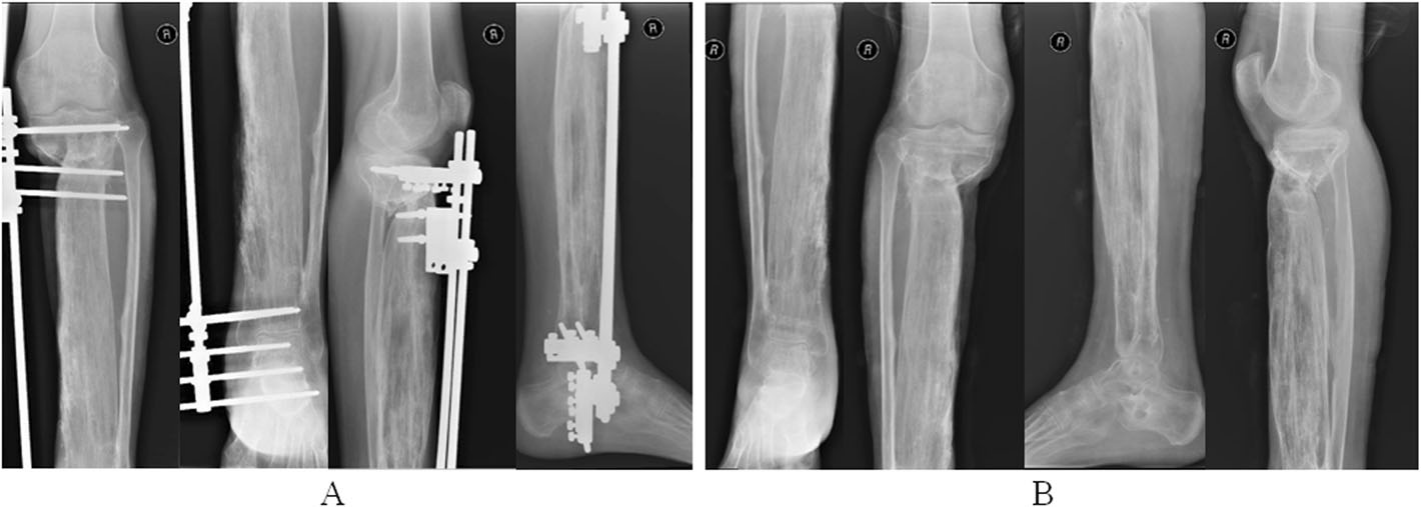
X-ray performed (a) 40 months after corticotomy and (b) following the removal of the external fixator after complete union at the docking site and bone consolidation
